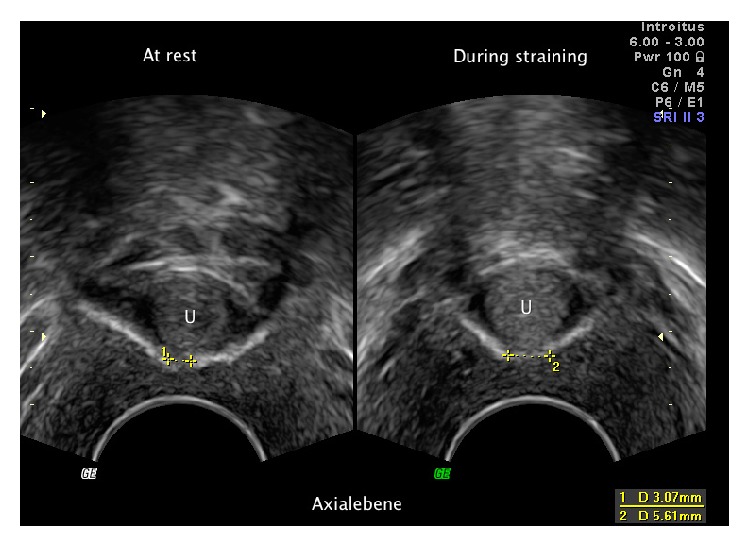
Pelvic floor ultrasound examination in axial plane. The sling was suburethrally split. Left: distance between both sling ends at rest. Right: during Valsalva manoeuvre.
